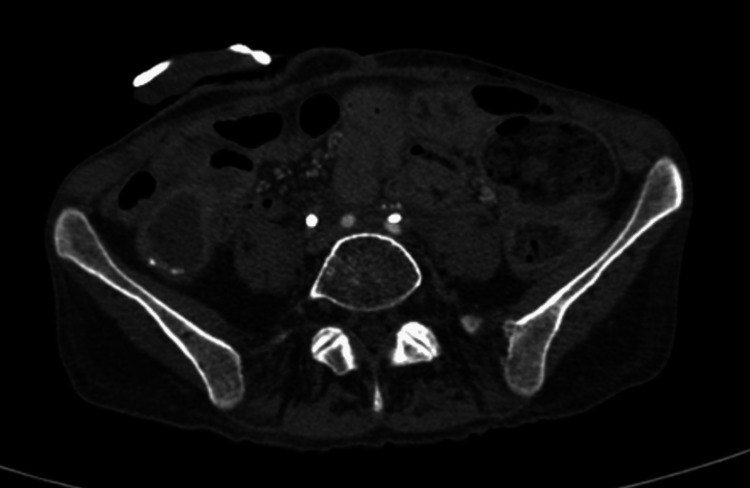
Contrast-enhanced CT (arterial phase). There is no plane between the ureteric stent and the left iliac artery.
